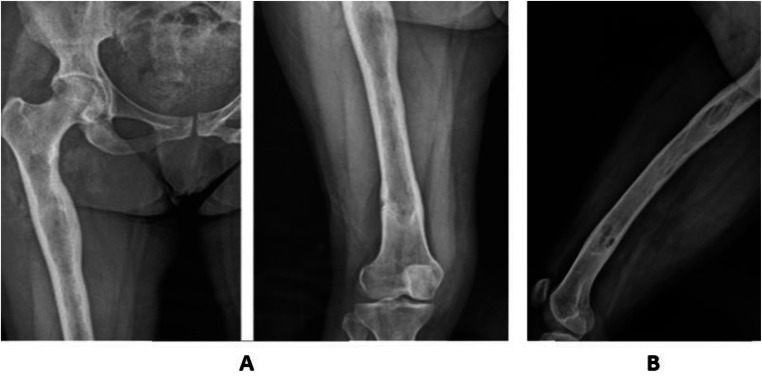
Standard Radiograph : F(A) /P (B) of the right lower limb. Extensive diaphyseal widening of the femoral bone associated with thickening of the cortical bone.
